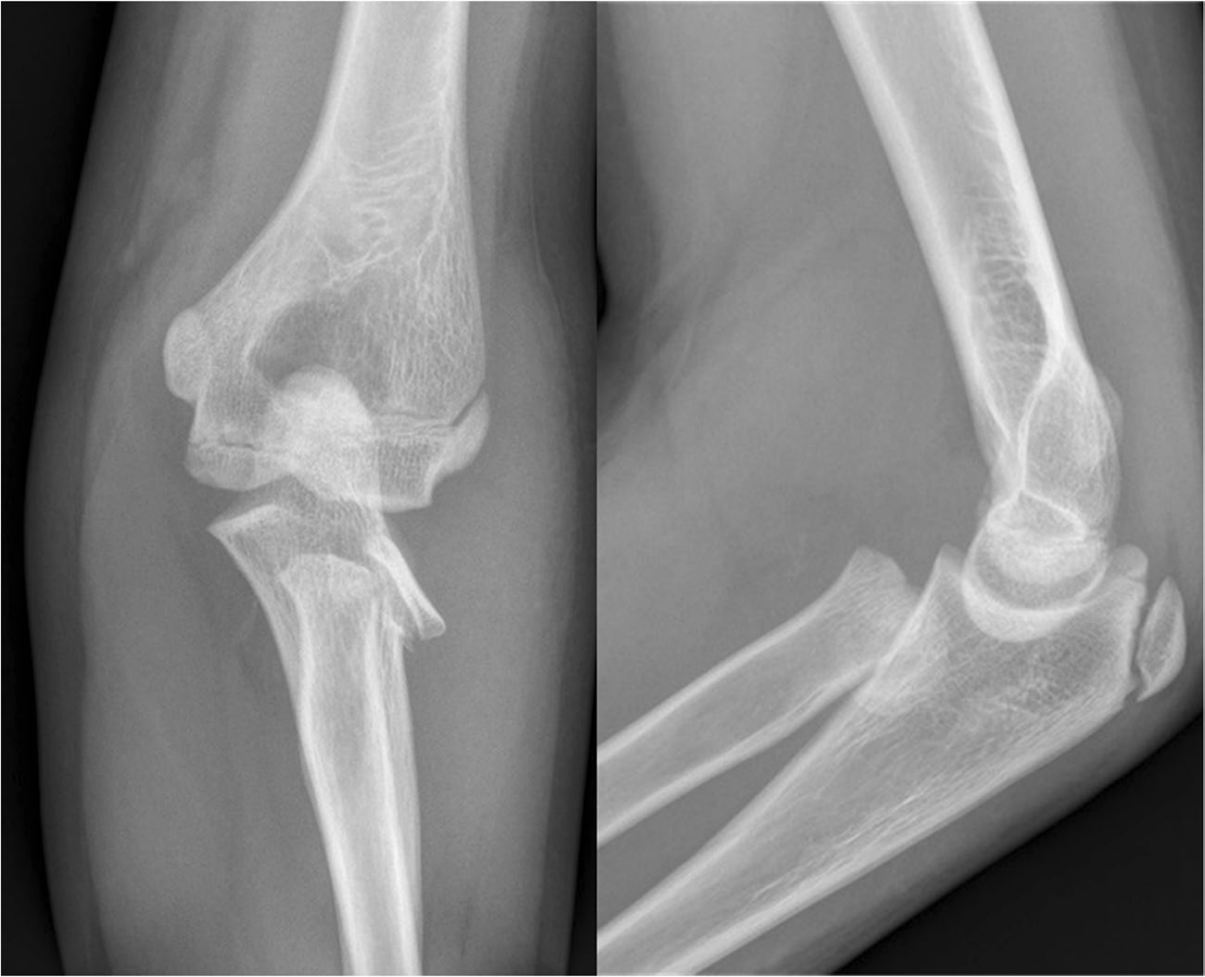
AP and L view radiograph of the elbow with radial neck fracture in a skeletally immature male
